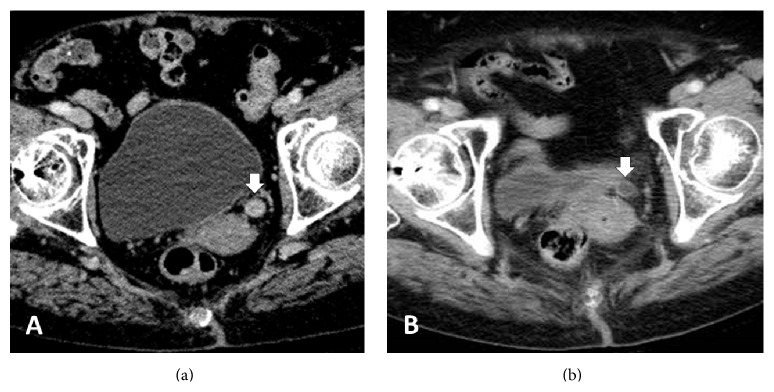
Contrast-enhanced CT scan of the patient. (a) A CT scan showed the tumor as enhanced thickness of the wall at the distal ends of the left ureter before the treatment (see arrow). (b) The enhanced thickness of the ureteral wall improved 3 months after SBRT.
